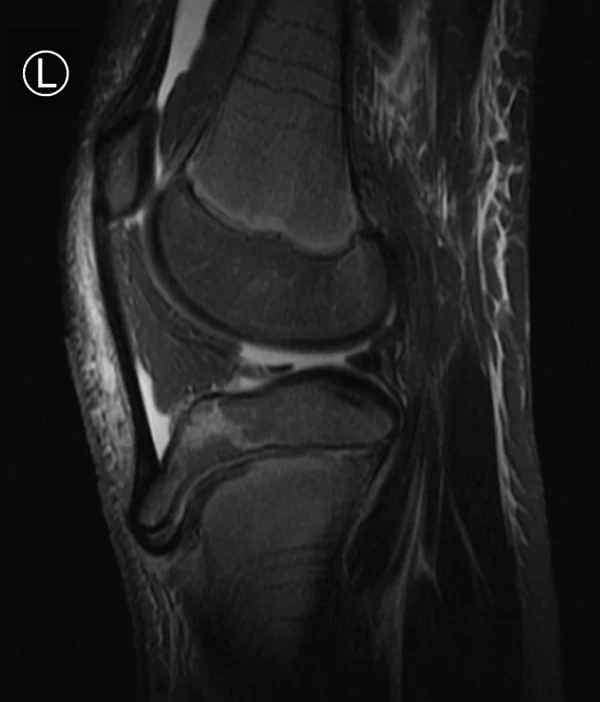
Left knee sagittal T2 MRI.
